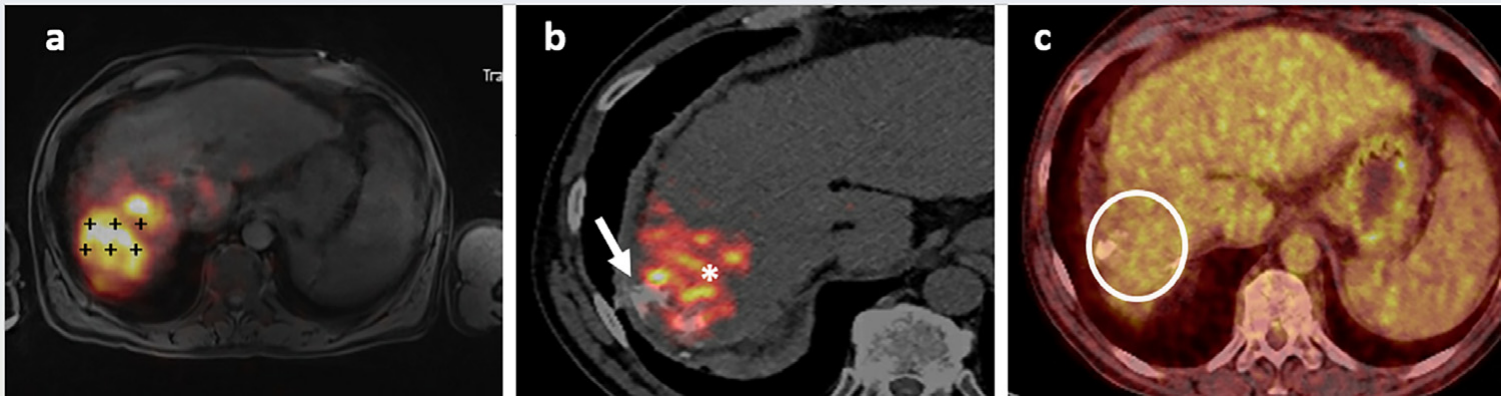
A: PET-MRI axial image showing a HCC of the hepatic dome (black crosses). B: ^90^Y PET-CT image after TARE and TACE showing the accumulation of ^90^Y in the inner part of the lesion (white asterisk) and the Lipiodol hyperattenuation of the outer part of the tumor, treated by TACE through the diaphragmatic artery vessel (white arrow). C: (^18^F)-FDG PET-CT performed 14 months after treatment, showing no residual tumor in the treated area (white circle).
